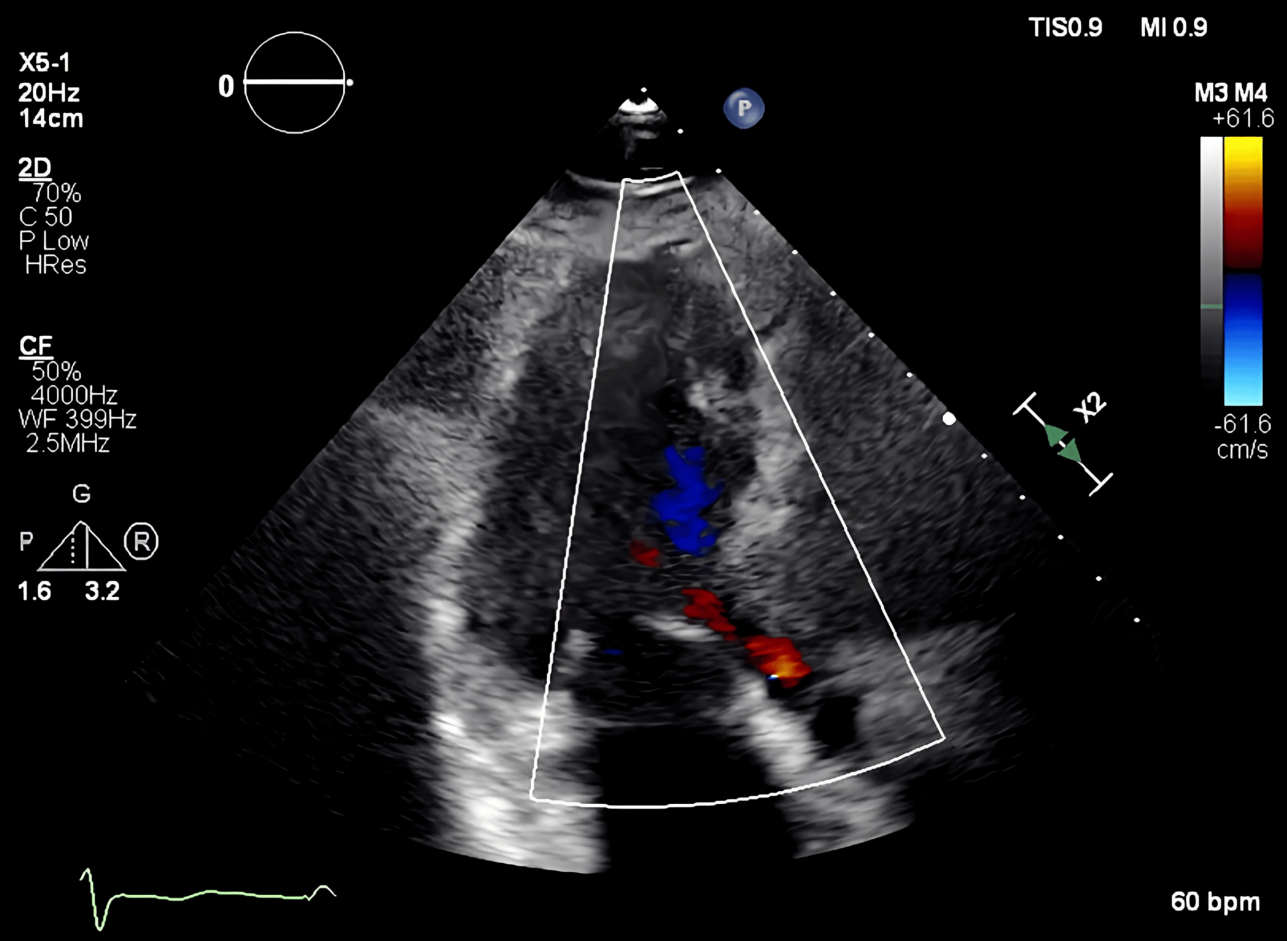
Follow-up echocardiogram Follow-up echocardiogram 1 year after surgery shows no signs of recurrence of subvalvular aortic stenosis and aortic regurgitation.
